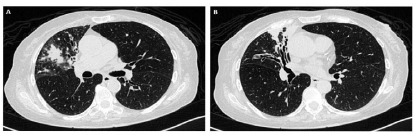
Female patient, 70 years old, diagnosed with bronchiectasis associated with Mycobacterium abscessus. In A and B there is a cut axial CT scan of the chest with thickening of the bronchial walls, especially in the right upper and middle lobe associated with foci of consolidation and irregular focal opacities.
